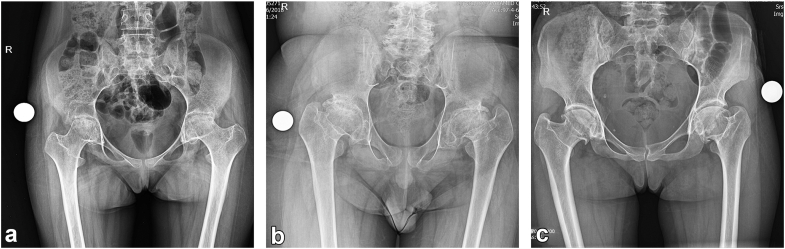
Preoperative anteroposterior pelvic X-rays of 3 patients: (a) a 17-year-old female, (b) a 16-year-old male, and (c) a 31-year-old female. Acetabular protrusion is evident in the left hip of case (a). Coxa profunda is observed in both hips of case (a) and the left hip of case (c). Coxa vara, trochanteric overgrowth, and a marked decrease in medial offset are present in both hips of case (b) and the left hip of case (c).
